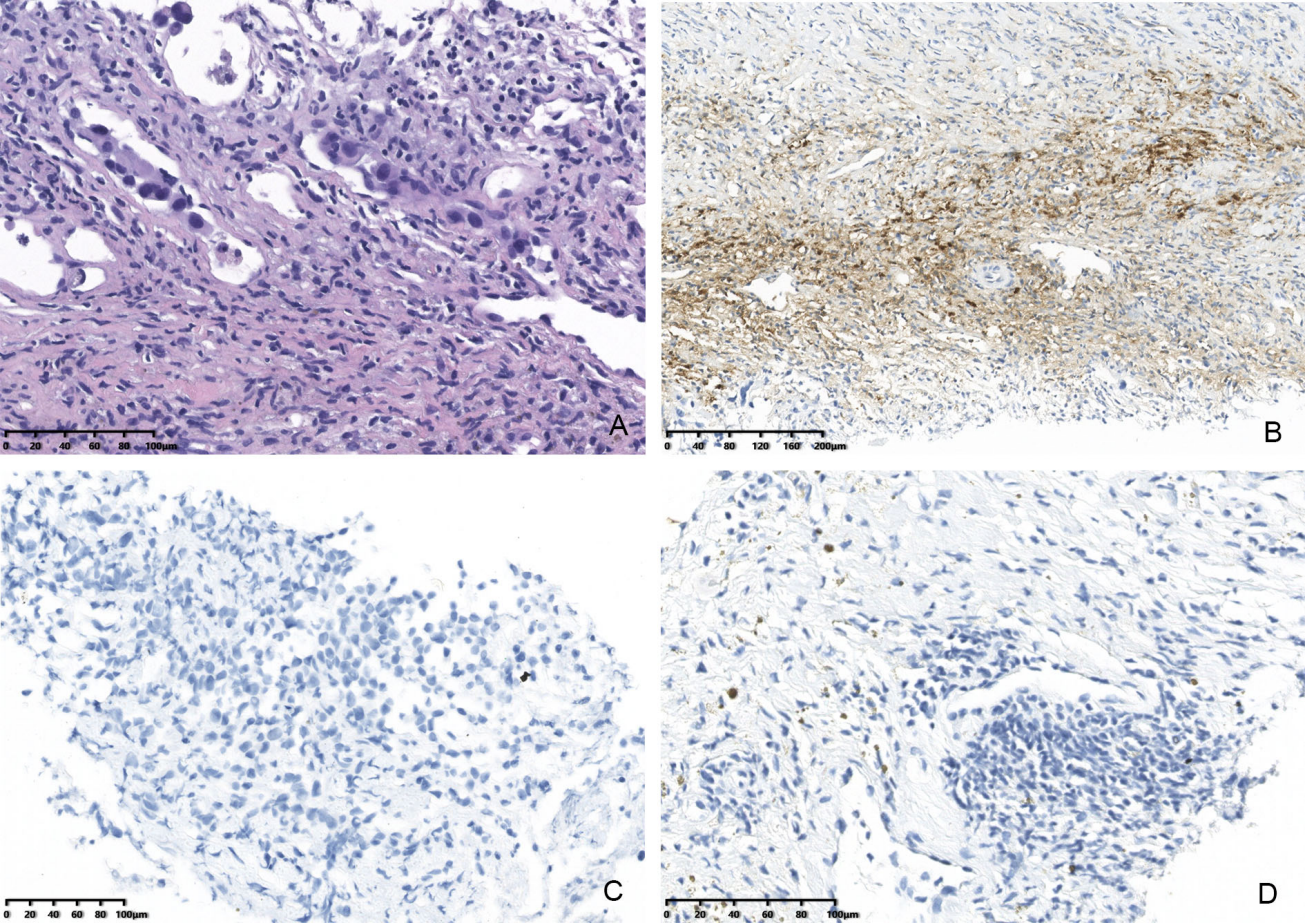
Computed tomography (CT) images showing that the size of the lesions had decreased. (A) Before treatment with dabrafenib and trametinib. (B) Dabrafenib and trametinib for 5 days. (C) Dabrafenib and trametinib for 19days. (D) Dabrafenib and trametinib for 50days.
